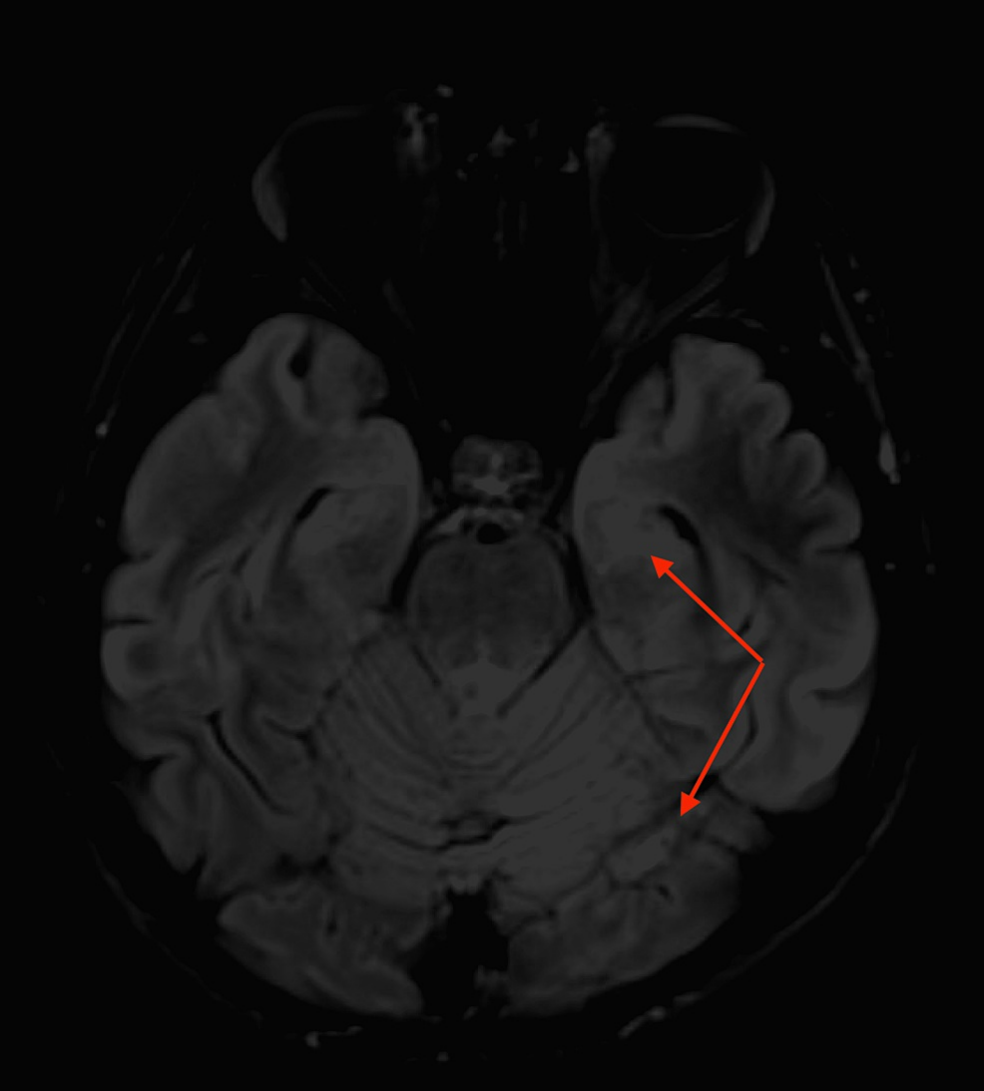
MRI of the brain. Red arrows pointing to lesions concerning possible acute embolic infarctions versus brain metastases
